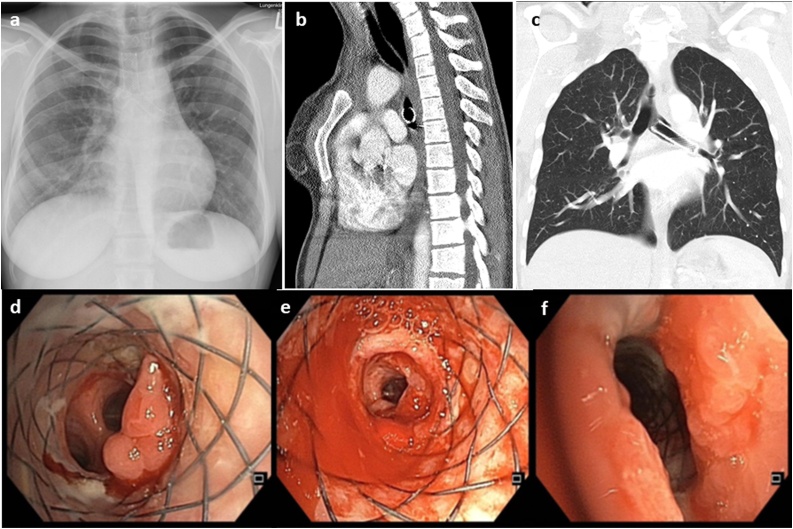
Stent and Pectus excavatum. a) Chest X-ray with a y-stent and bilateral streaky infiltrates and a right sided synostosis of the 5th and 6th rib 2012. b) Sagittal CT-plane demonstrating the pectus deformity of the sternum 2015. c) Coronary CT-plane of the lung with a covered metal stent 2017. d–f) Bronchoscopic views of the last stent with granulation tissue formation at the proximal and distal stent ends and chronic inflammatory changes of the bronchial mucosa.
